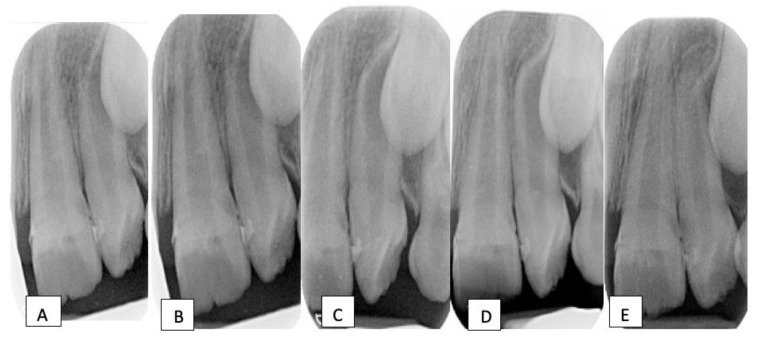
Radiographic evaluation of 11 & 21; (A) 2 weeks after the surgery (immediate post-operative review), (B) at 3-month, (C) at 6-month, (D) at 12-month, (E) at 24-month recall visits. Notice the continued root development and the apical closure in the hypoplastic teeth.
